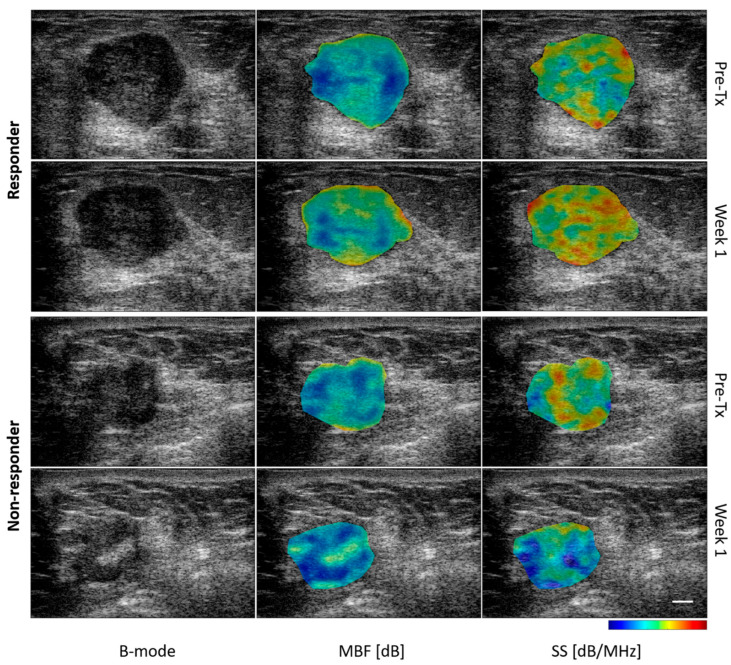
Representative B-mode, mid-band fit (MBF) and spectral slope (SS) parametric images at baseline (pre-Tx) and after the first week of treatment for one patient from responder and non-responder group. The scale bar in the ultrasound images represents 5 mm. The colour bar represents the scale for the MBF of −10 to 30 dB, and for SS parameter of −6 to 2 dB/MHz.
